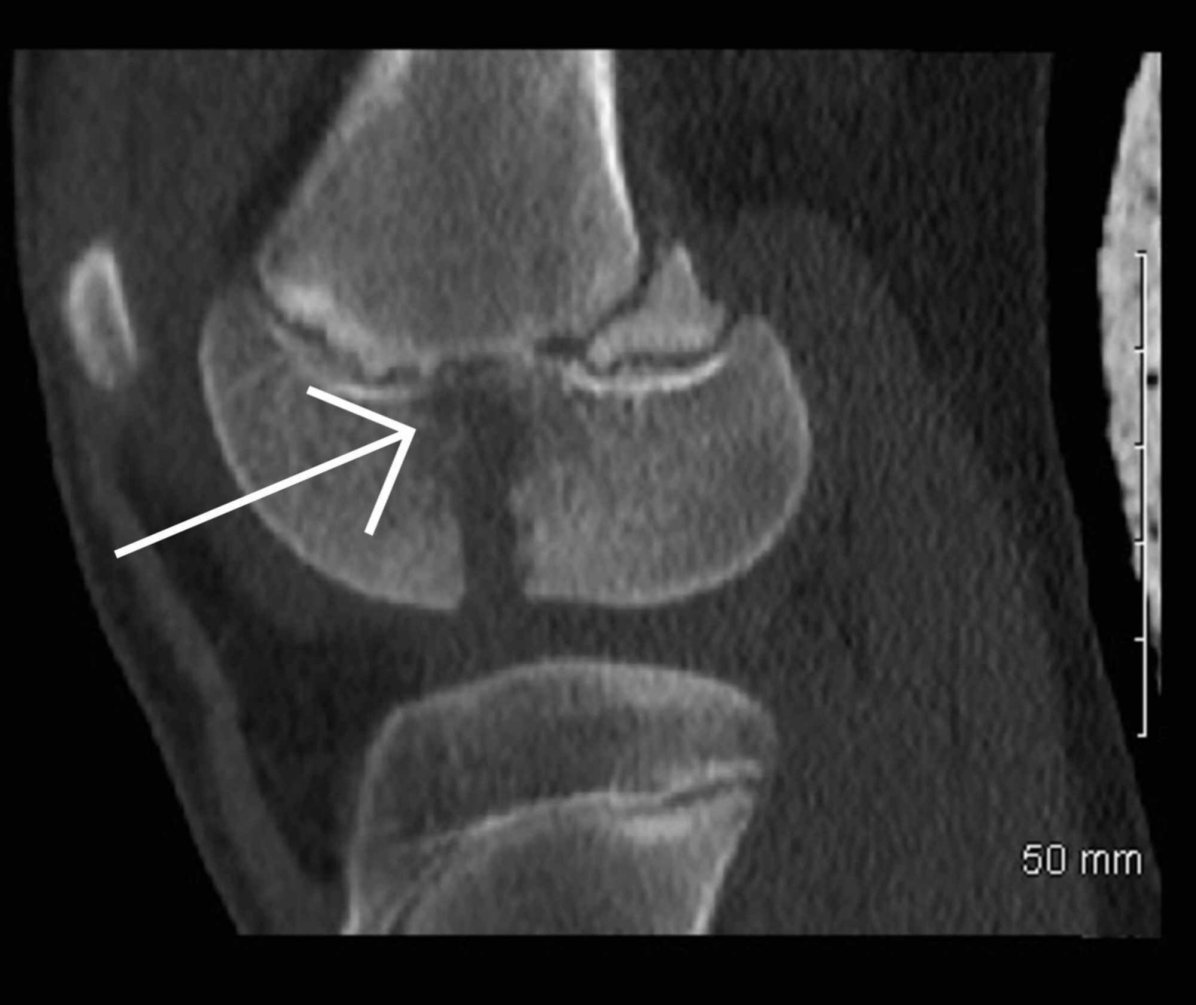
Sagittal view of computed tomography scan of right knee post-injury
